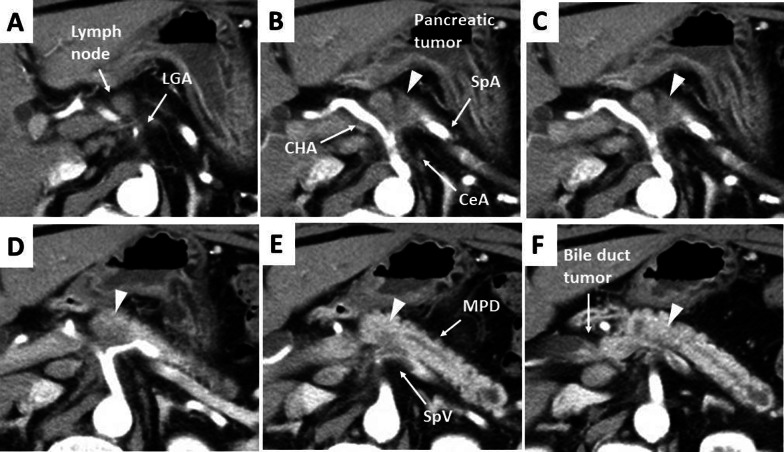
Abdominal contrast computed tomography (CT) findings at initial diagnosis. A The left gastric artery (LGA) was surrounded by soft tissue. Swollen regional Lymph node was adjacent to the pancreatic tumor. B–D 40-mm-sized hypovascular mass in the pancreatic body that was radiologically consistent with pancreatic ductal adenocarcinoma (arrowhead) showing wide abutment the common hepatic artery and the splenic artery (SpA). C Hazy attenuation connected to the tumor was seen on the Celiac artery. D Contour irregularity was seen on SpA and focal narrowing was seen on the splenic vein (SpV). E The main pancreatic duct (MPD) was slightly dilated in the distal side. F the lower bile duct tumor was enhanced
